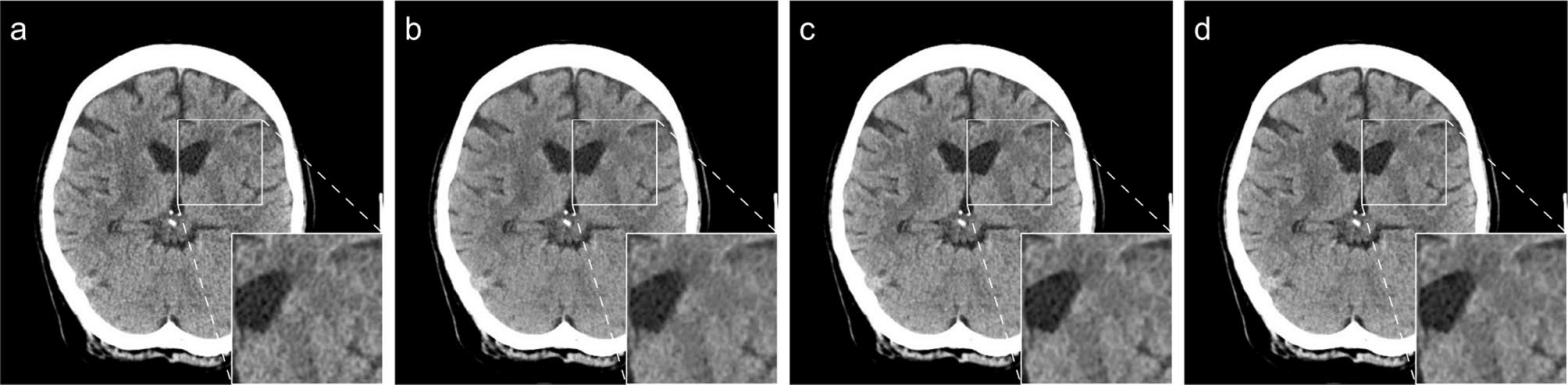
Example of four differently reconstructed and post-processed images from the same CT scan (Siemens SOMATOM Force, site I). In each figure, a section of the images is zoomed and shown in the lower right corner. (a) Image reconstructed with filtered-back projection (FBP). (b) Image reconstructed with filtered-back projection and post-processed (FBP + PS). (c) Image reconstructed with iterative reconstruction (I3). (d) Image reconstructed with iterative reconstruction and post-processed (I3 + PS).
